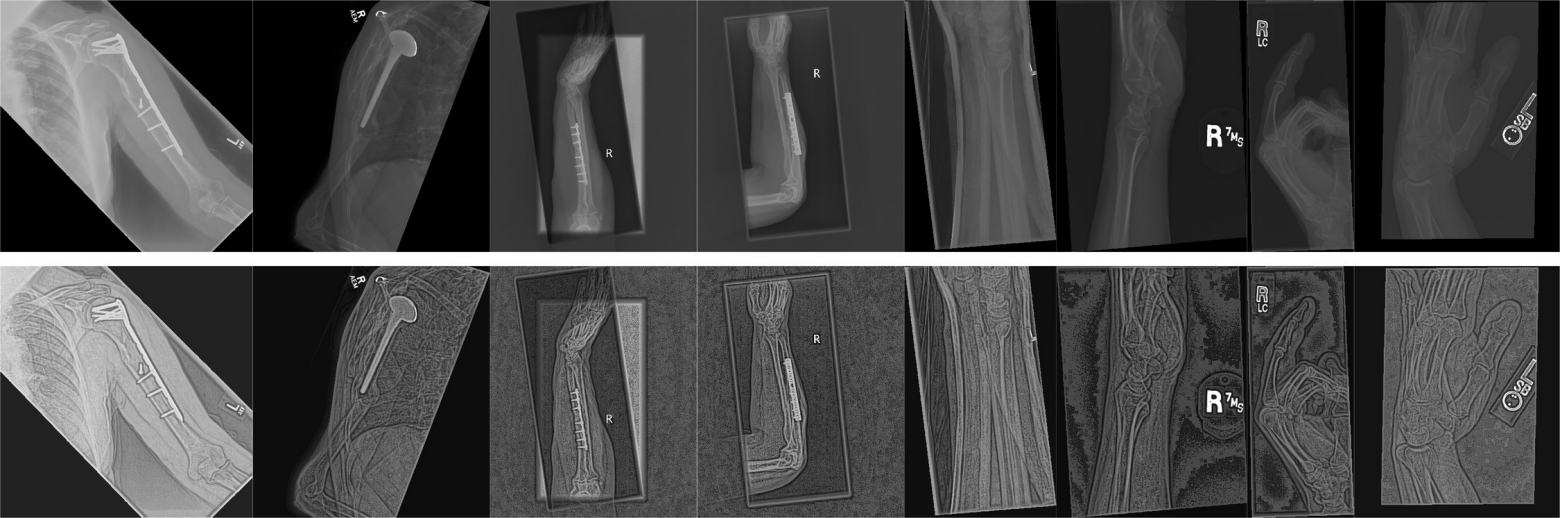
Generalization test of the proposed method on X-ray images of various body parts, including the humerus, forearm, wrist, and fingers, taken from the MURA dataset.
